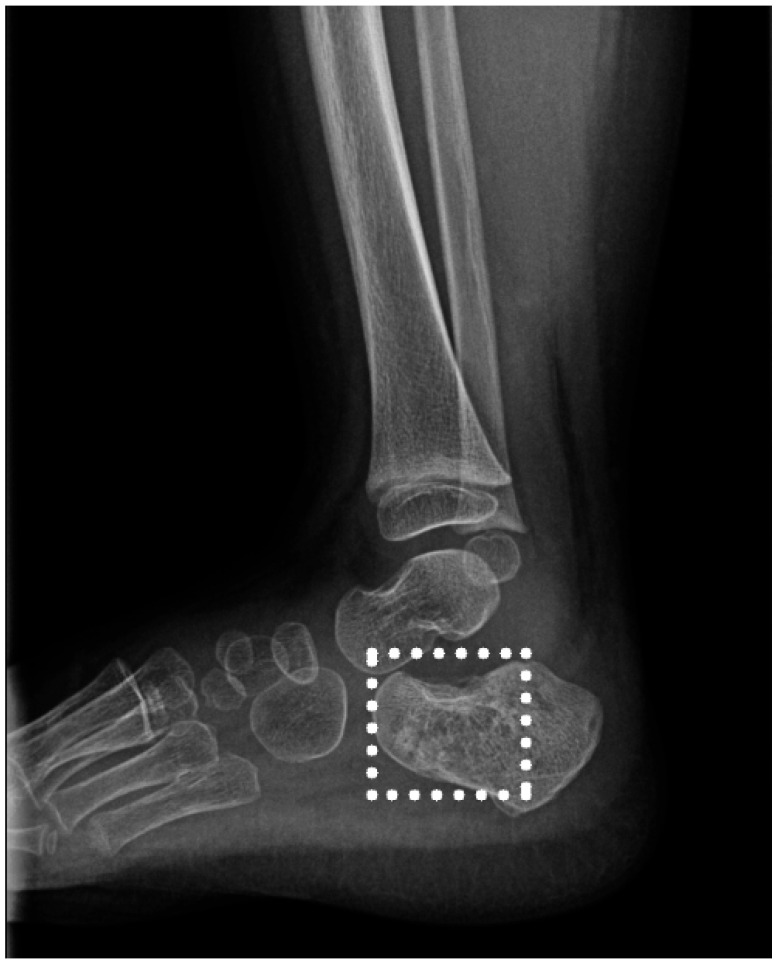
Right ankle X-ray: demineralization and irregular areas of osteolysis and osteocondensation at the level of the calcaneus. The square indicates the lession area.
